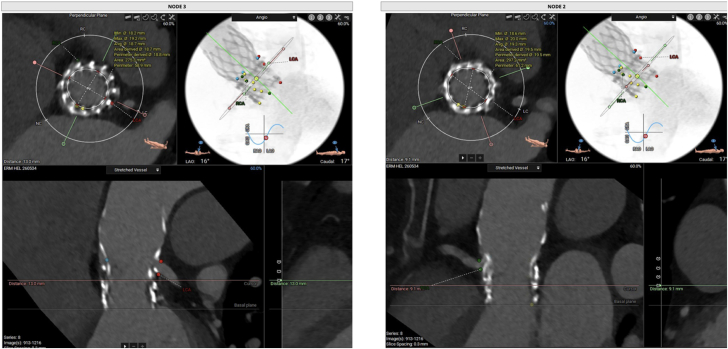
Computed Tomography Angiography-Based Measurements on the 23-mm Evolut Pro Nodes 2 and 3 The measurements demonstrated an area of 297.2 mm^2^ for node 2 and 275.3 mm^2^ for node 3.
